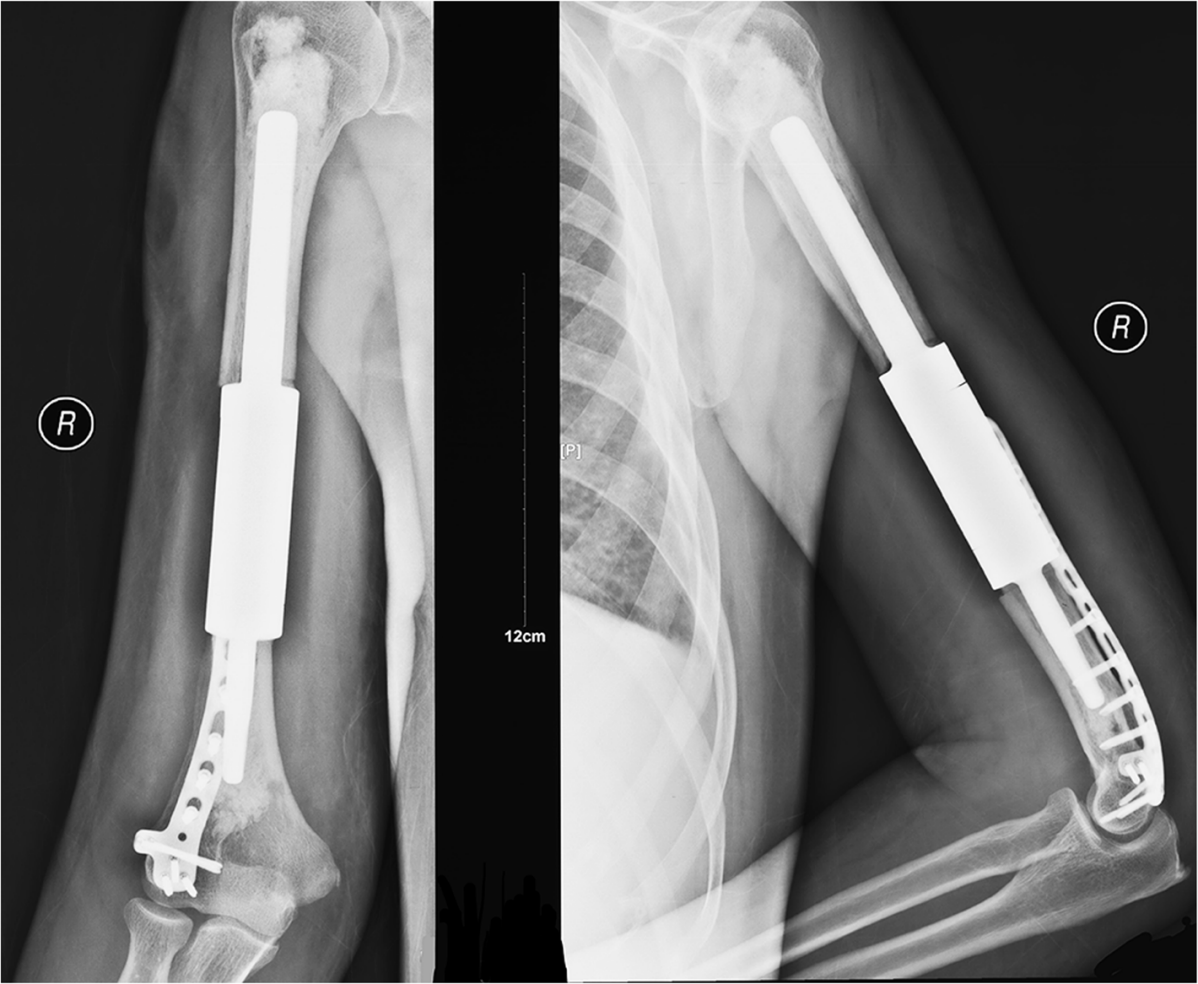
The postoperative X-ray film shows that an additional extracortical plate was applied when the fixation length was less than 5 cm
